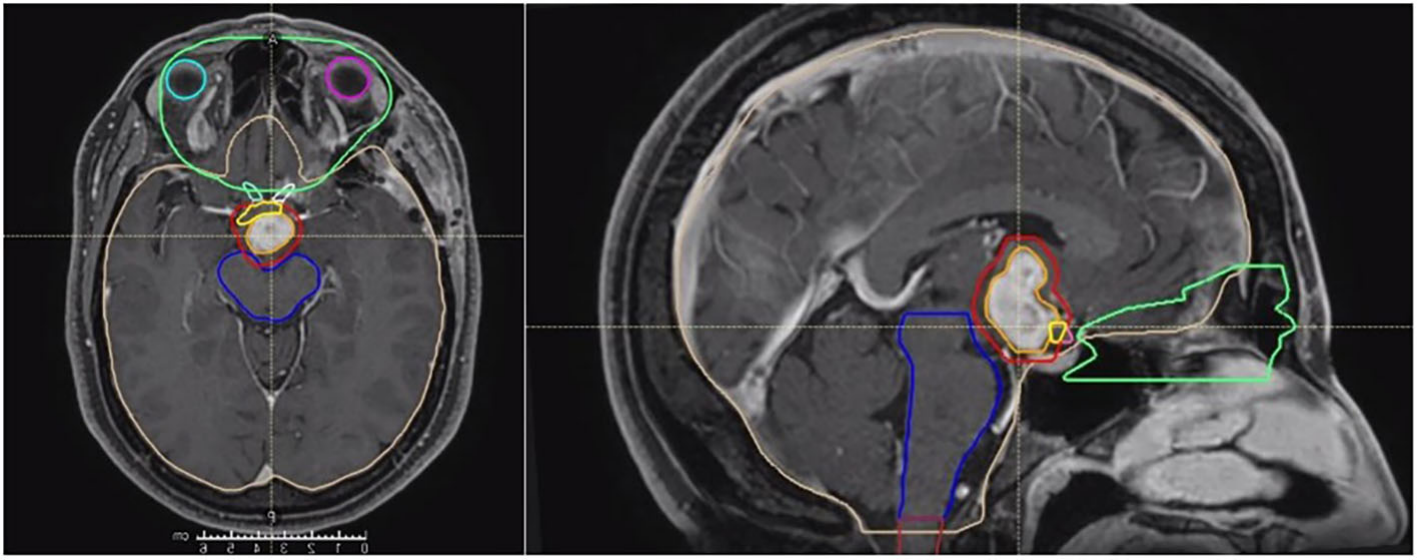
MRI imaging after surgery.
